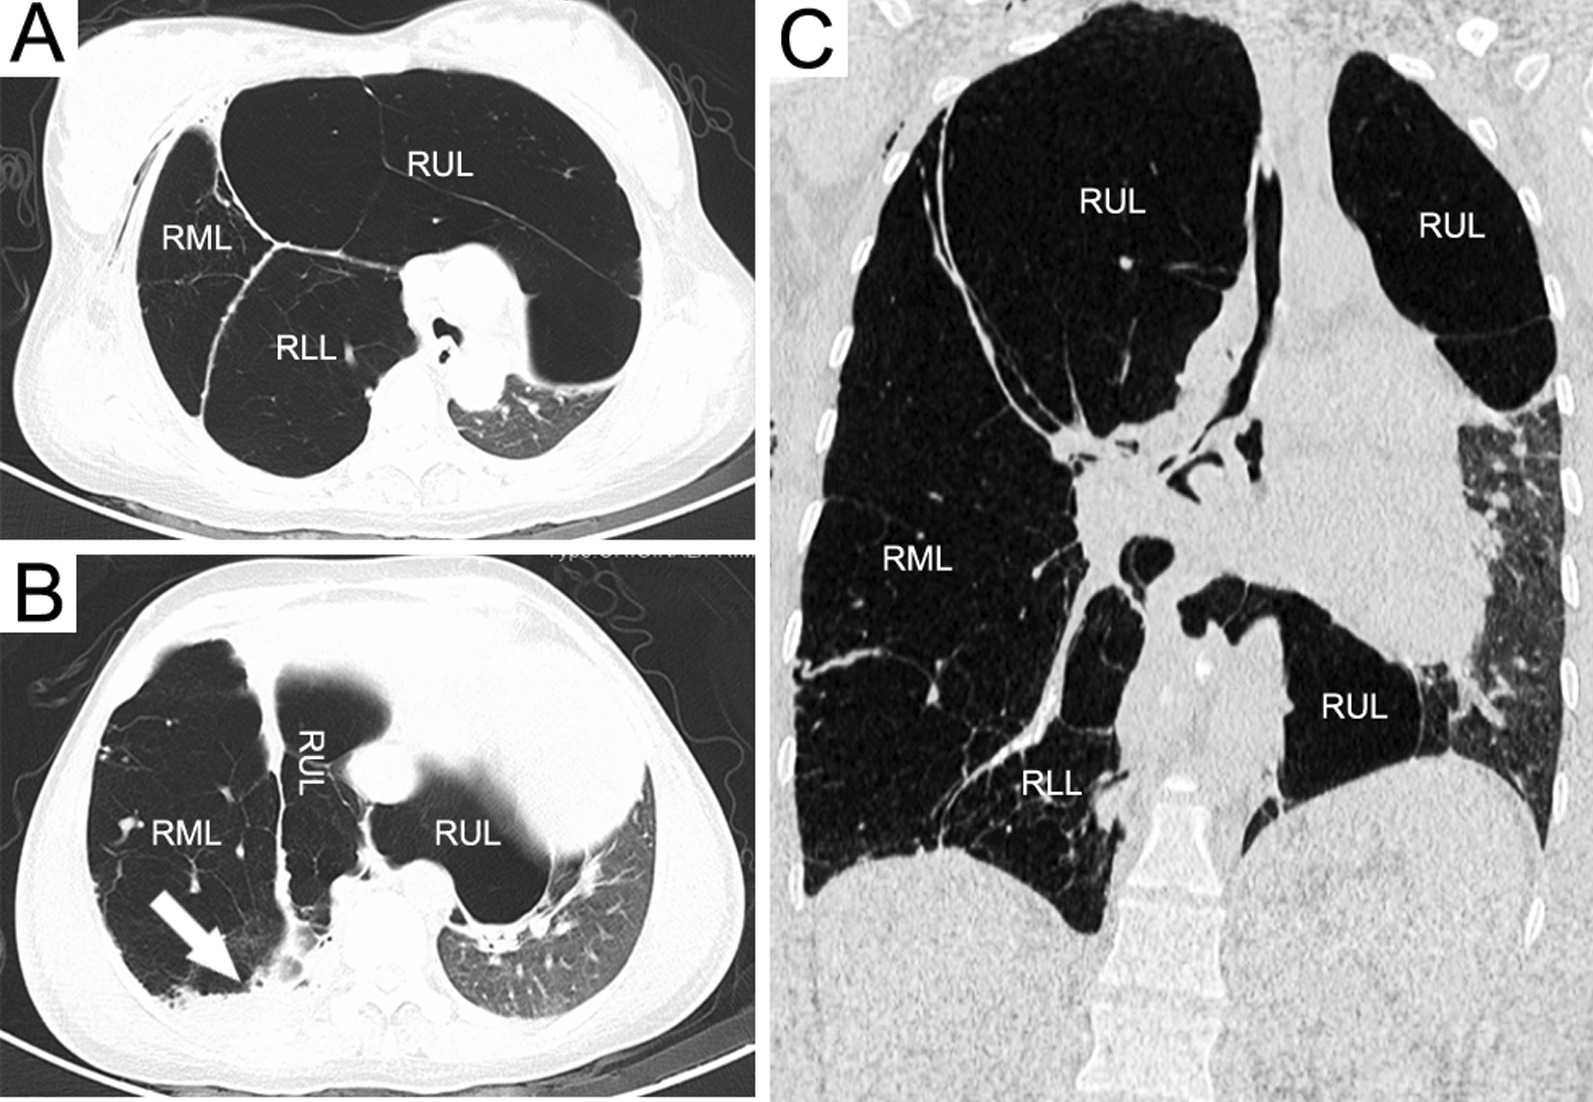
Chest computed tomography (CT) displaying emphysema of the right upper, middle and lower lobes resulting in adjacent atelectasis (arrow); hyperinflation of the right upper lobe crossed the midline, leading to a deviation of the mediastinal structure to the left hemithorax and severe compression of the left lung. A and B, Axial chest CT scans; C, Coronal chest CT scan. RUL, right upper lobe; RML, right middle lobe; RLL, right lower lobe
